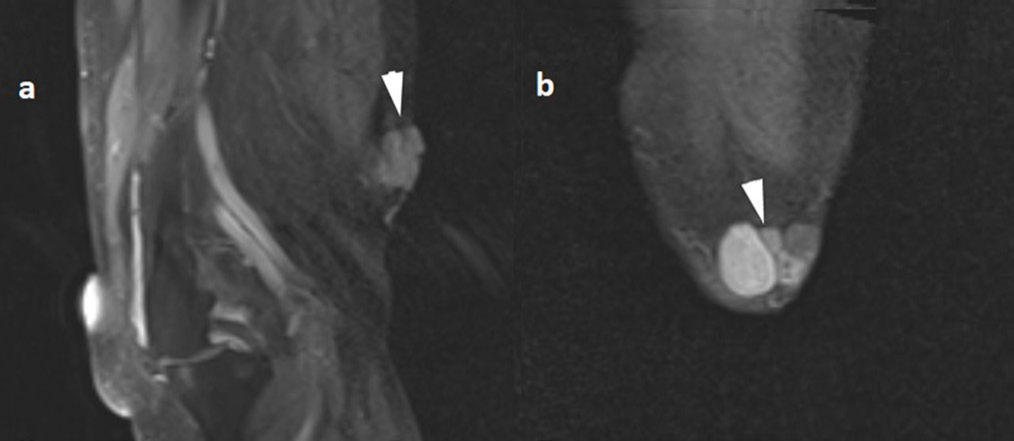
Coronal (FS) MRI (a) and sagittal (FS) MRI of the skin mass after administration of contrast.
